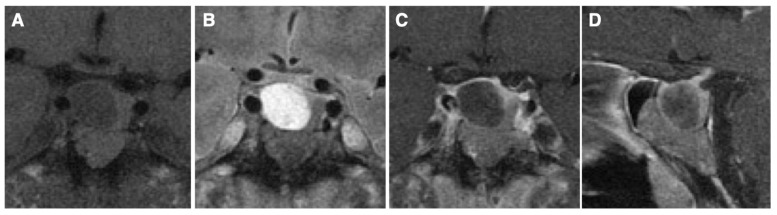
Magnetic resonance images: T1-weighted (A), T2-weighted (B), gadolinium-enhanced T1-weighted (C) in the coronal section, and T1-weighted image in the sagittal section (D), showing a 2.5 cm diameter macro-tumor with suspected cystic degeneration in the upper portion.
